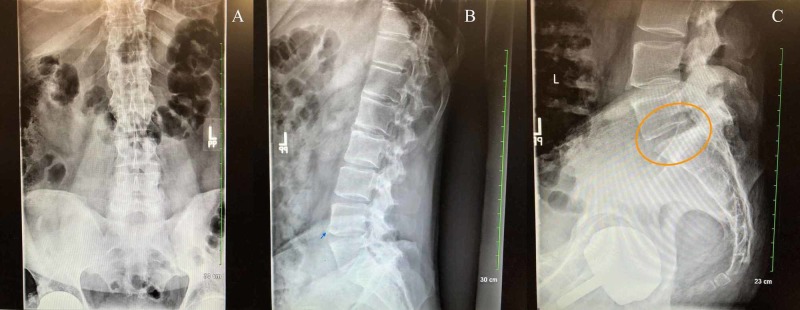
X-ray of the lumbar spine Findings: The heights of the lumbar vertebral bodies are normal. There are degenerative facet changes at lumbar spinal (L) levels 4 and 5 (L4-5). Mild narrowing of lumbar spine level 5 and sacral disc 1 space (L5-S1) (image C, orange circle). There is some anterior spurring of L4-L5 (image B, blue arrow) and at L1-L2. Remaining lumbar disks are well preserved. Impression: Degenerative changes as described above. Findings are most marked in the facets at L4 and L5. There is no evidence of vertebral fracture.
